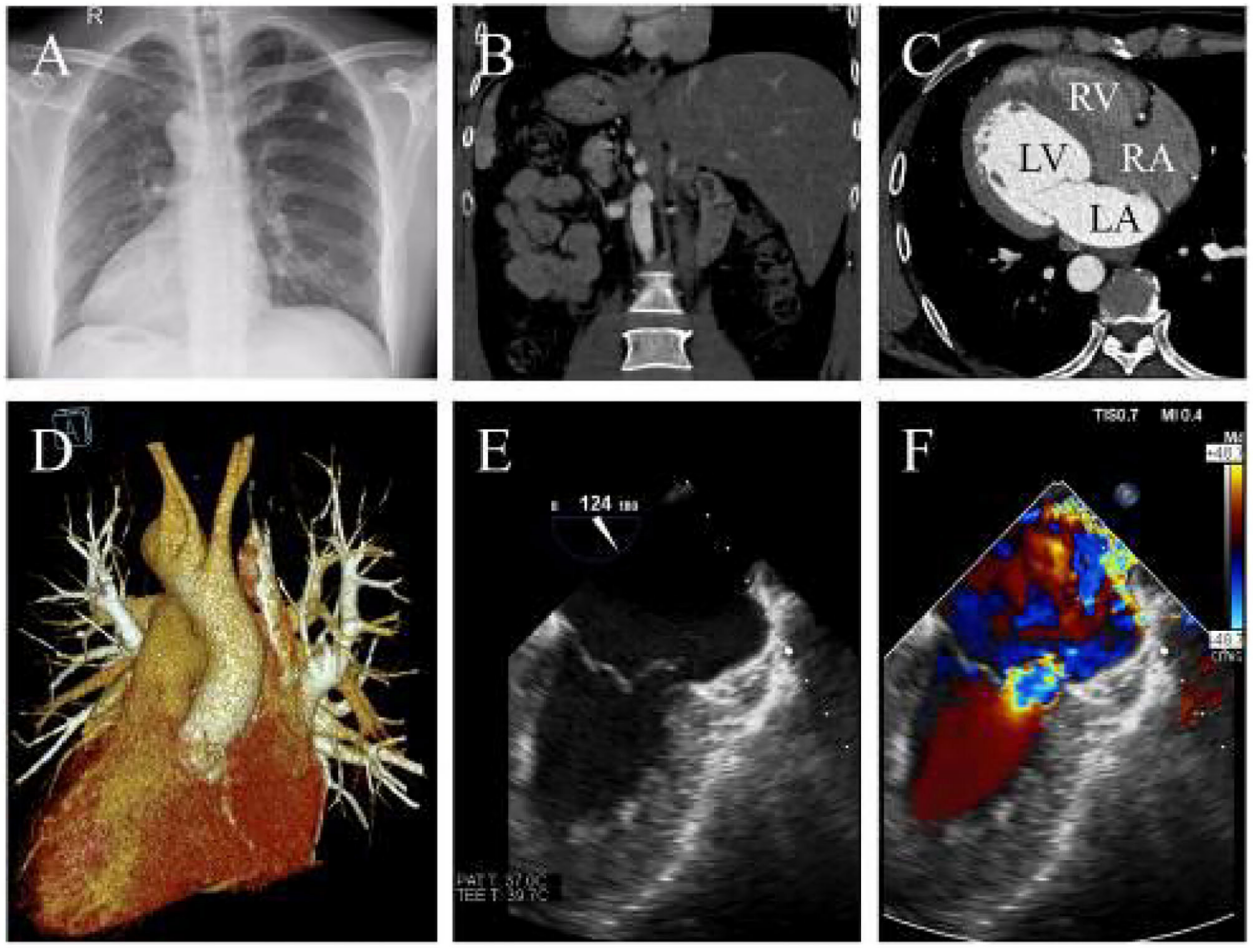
(A) X-ray indicates dextrocardia and cardiac enlargement. (B) CTA reveals transposition of the abdominal organs and (C) confirming L-loop ventricular orientation and concordant atrioventricular connections. (D) Three-dimensional CTA shows normal great artery development and connections. (E) The transesophageal echocardiography shows mitral chordae rupture and (F) severe mitral regurgitation (LA, left atrium; LV, left ventricle; RA, right atrium; RV, right ventricle).
